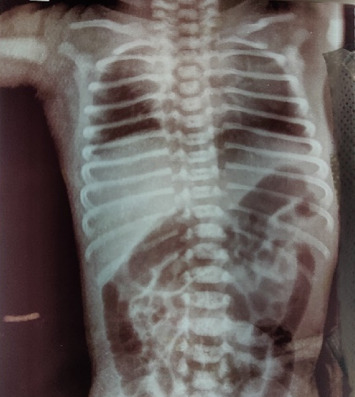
X-ray PA view showing absent sternum and collapse of the left upper lobe.
